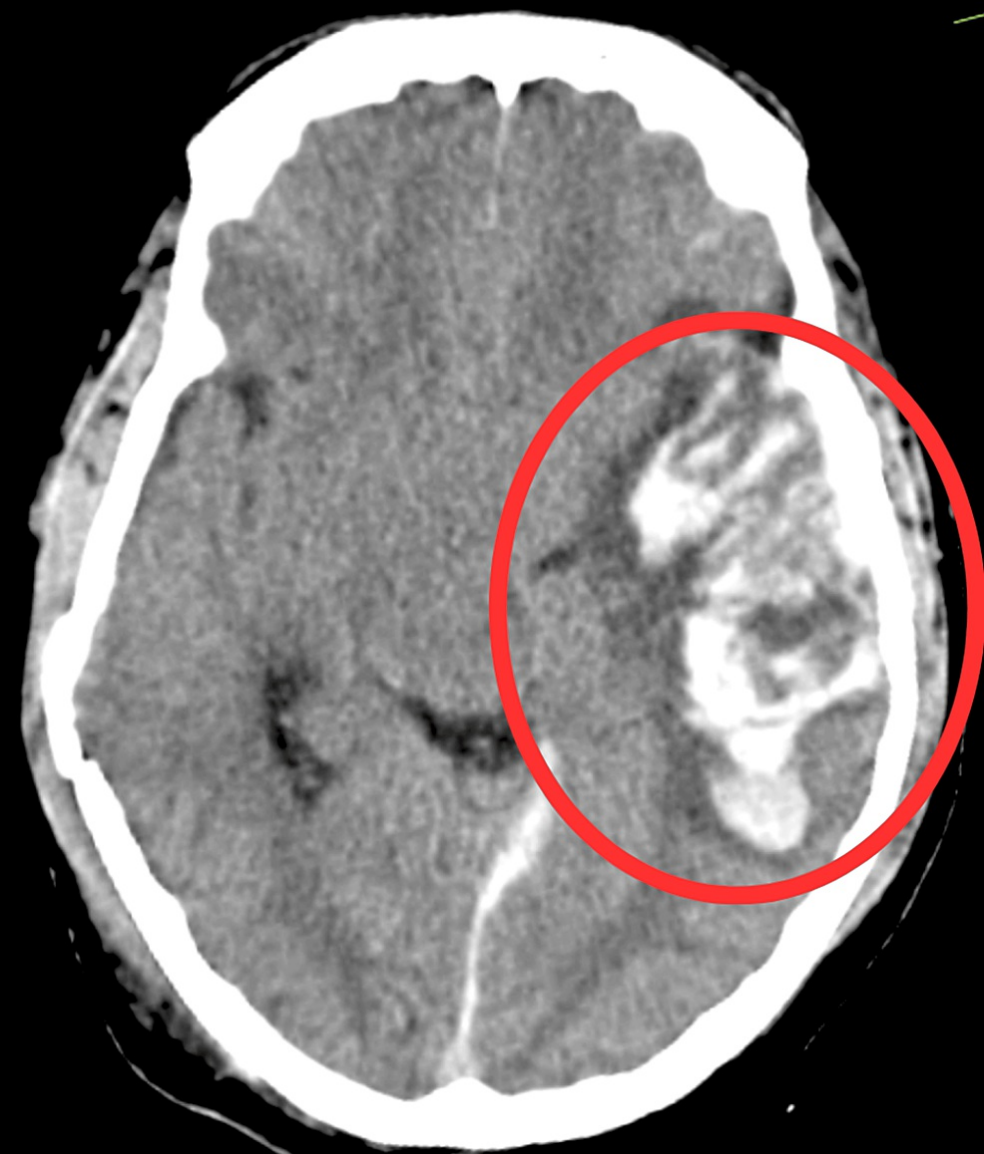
Pre-operative computed tomography scan The red oval shows hemorrhagic contusion in the left temporo-parieto-frontal region with intraventricular extension and mass effect
